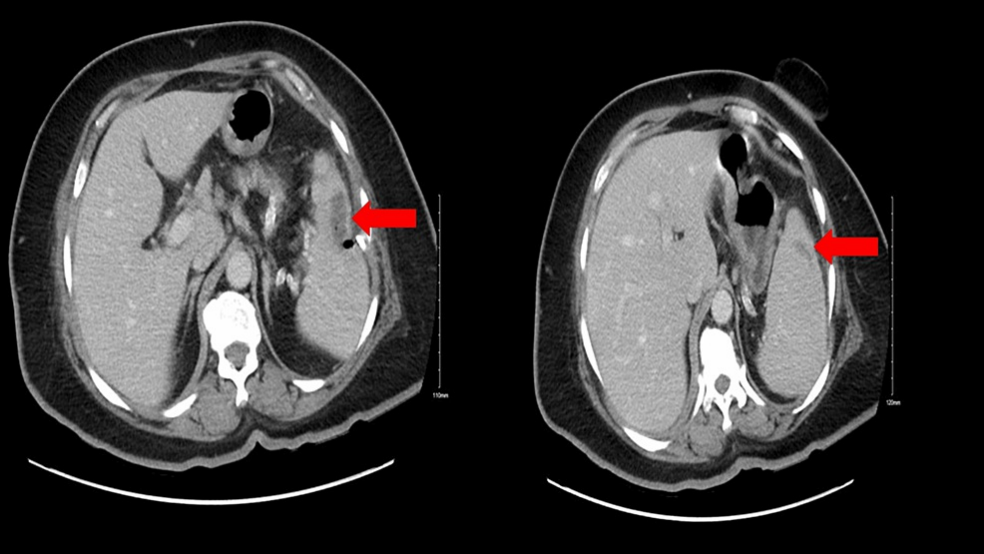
Follow-up CT scan performed six months after the discharge showing almost-complete resolution of the splenic abscess (red arrows) CT: computed tomography
